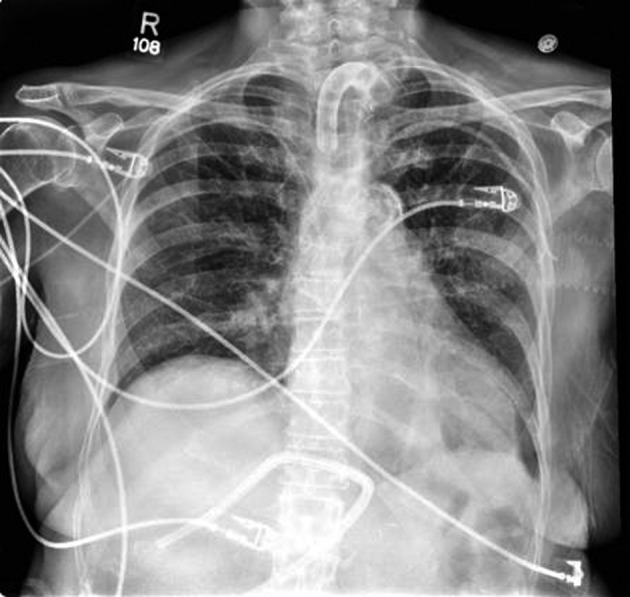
Chest X-Ray following ligation of thoracic duct and removal of drains, POD12.
